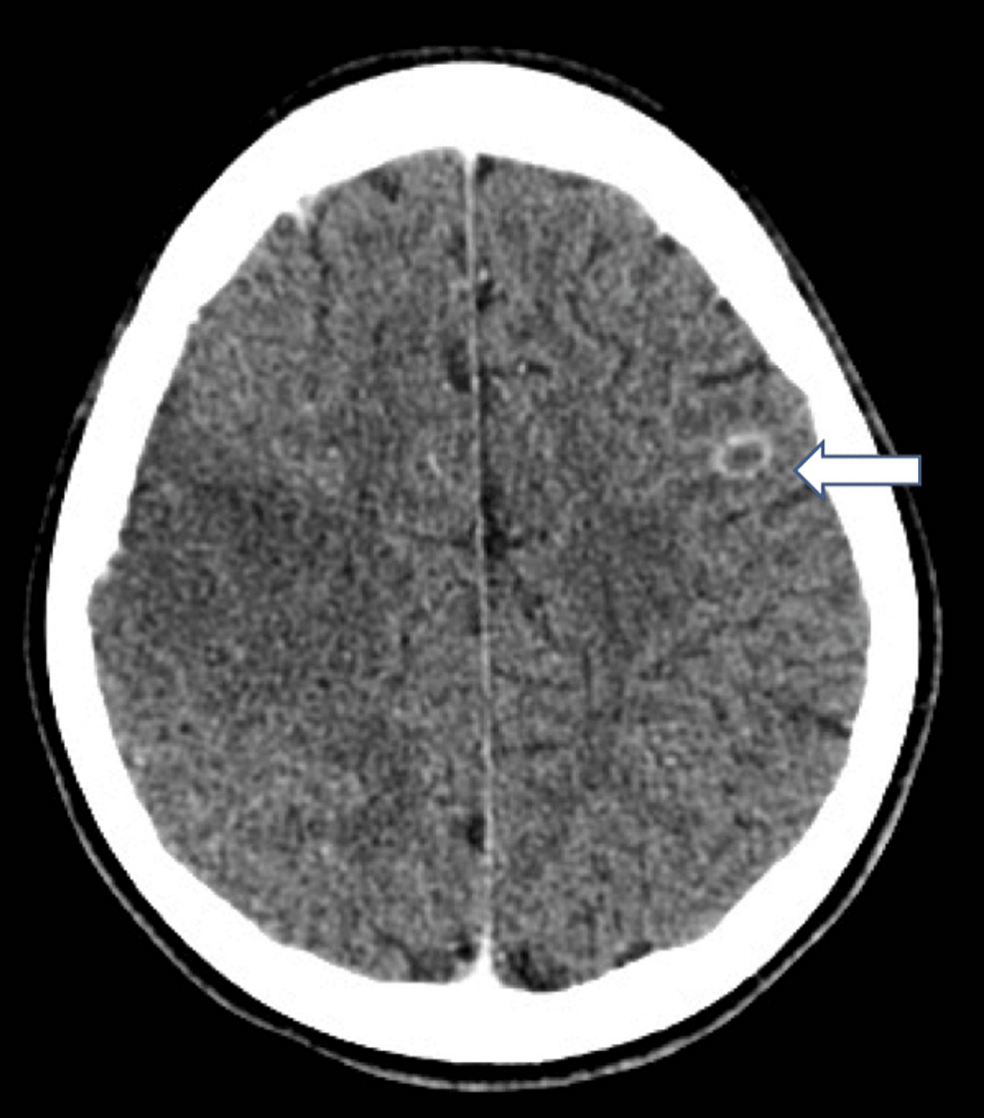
CT brain in Case 3 showed a ring-enhancing lesion in the left frontal lobe suggesting abscesses.
